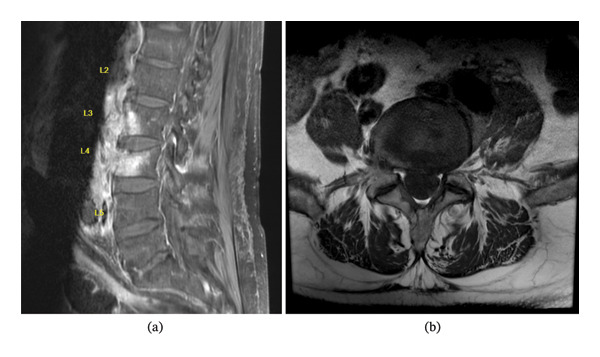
Lumbar spine MRI: sagittal view (a) and axial view (b).
